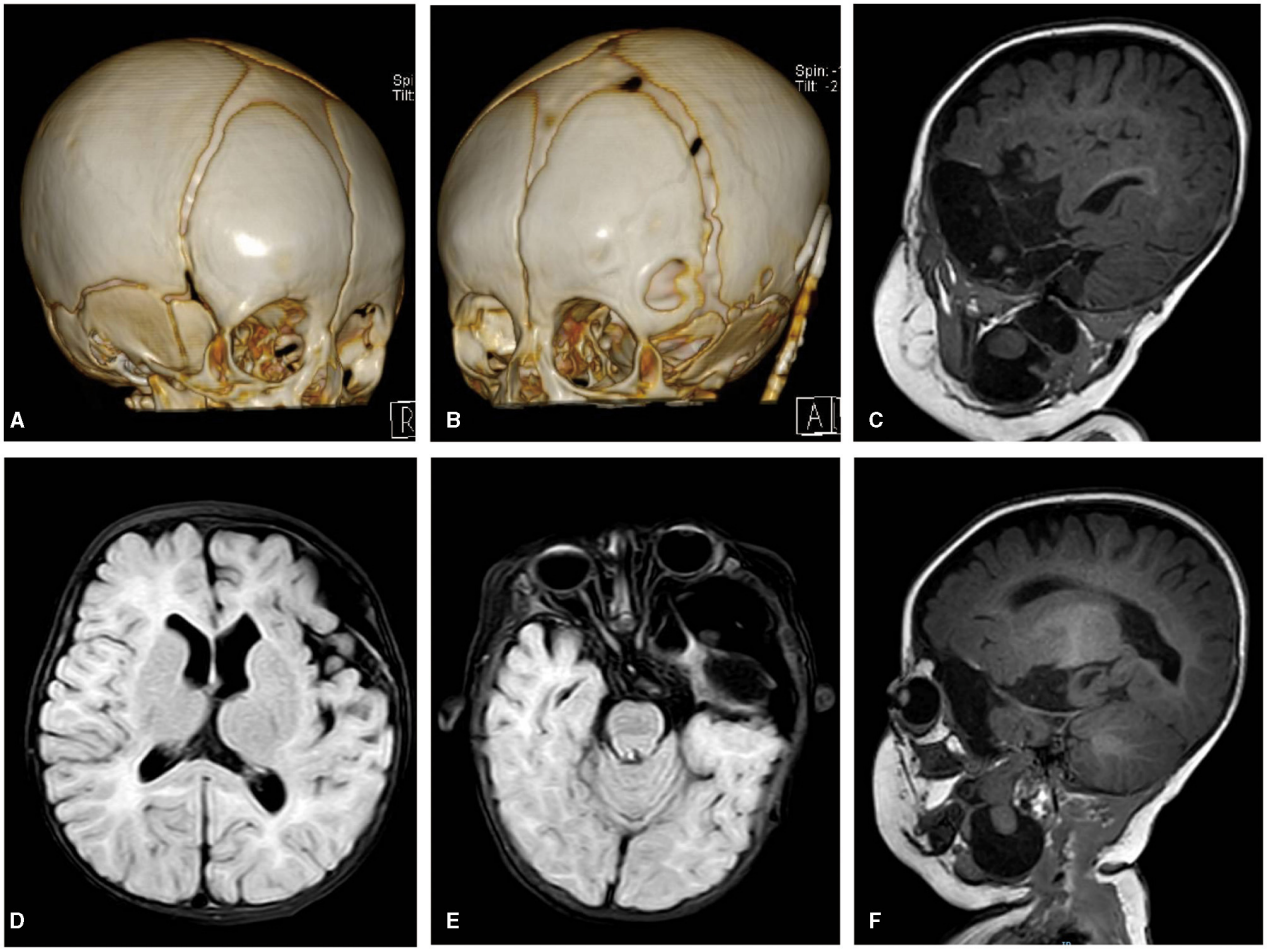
(A) A 3-D CT image of the skull's right frontal view showing the right GWS ossified well. (B) A 3-D CT image of the skull's left frontal view showing there was a defect on the outside of the left GWS and the tube of the CP shunt. (C–F) FLAIR axial and sagittal T1WI images showing a cystic lesion with CSF signal intensity in the left middle cranial fossa were significantly reduced, and correspondingly, the intracranial compression structure was also significantly relieved compared with the preoperative period. There was a partial loss in the left insular opercula and the temporal pole. The brain tissue signal intensity lesion in the cyst still communicated with both parts of the intracranial and neck through GWS.
